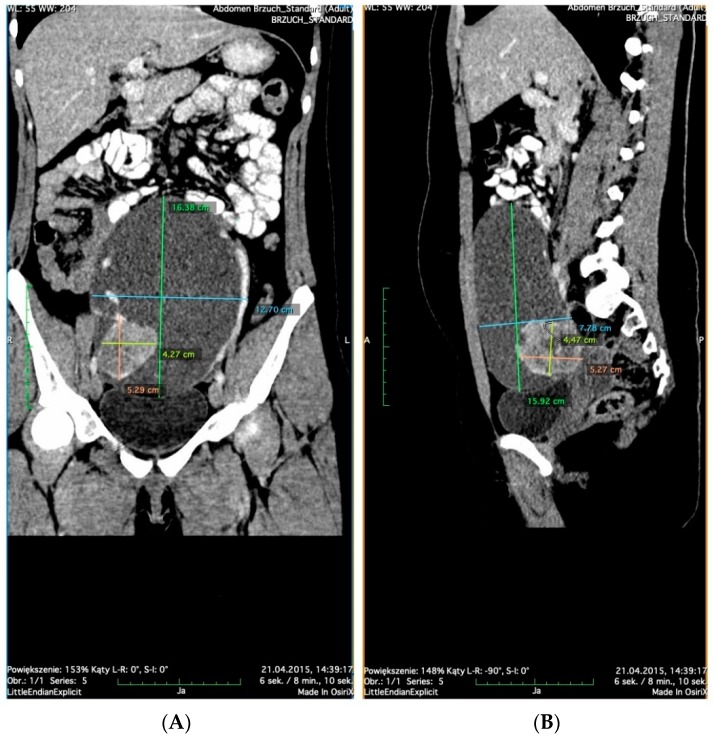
CT scans of the abdominal cavity and pelvis, which revealed a vast cystic-solid lesion: (A) coronal CT; (B) sagittal CT.
